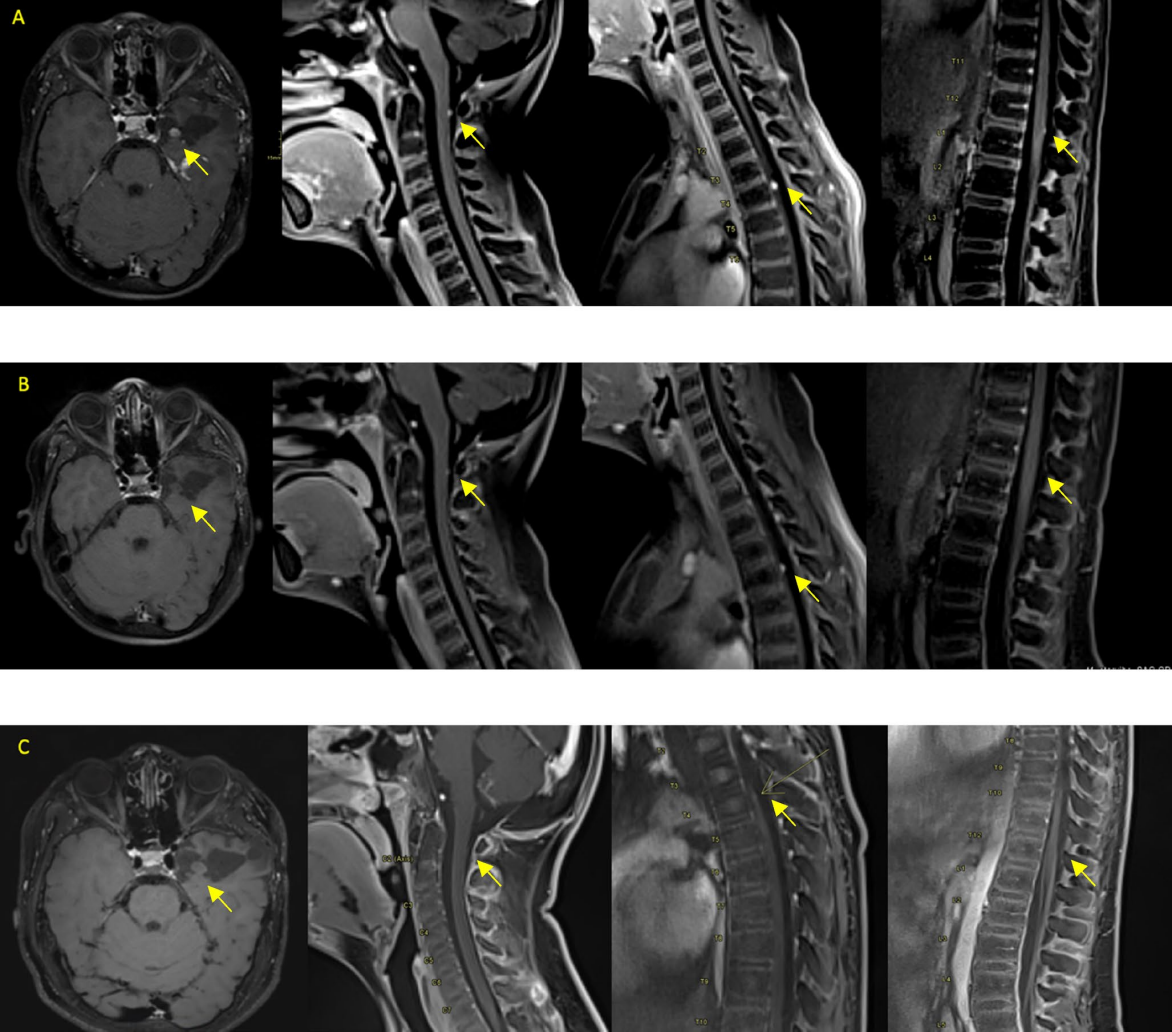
Legend, from left to right. Axial T1‐weighted brain MRI after gadolinium administration and sagittal T1‐weighted spinal MRI after gadolinium administration, performed with a 1.5 Tesla MRI scanner. (A) Baseline, prior to target therapy Brain MRI: 20 mm, 8 × 6 mm, and 1.5 mm contrast‐enhancing nodules at the margins of the resection cavity along with diffuse intracranial leptomeningeal enhancement Spinal MRI: 3.5 mm contrast‐enhancing lesion at cervical vertebrae 2, 5.7 mm at thoracic vertebrae 3, and non‐measurable lesion at thoracic vertebrae 12. (B) After 3‐months of target therapy, there was a lowering of contrast enhancement for all nodules and disappearance of the two smaller lesions. (C) After 24 months of target therapy, stability in residual size with inconsistent contrast enhancement in the bigger nodule, while the secondary lesions are almost undetectable. Nonspecific contrast enhancement of the cerebellum.
